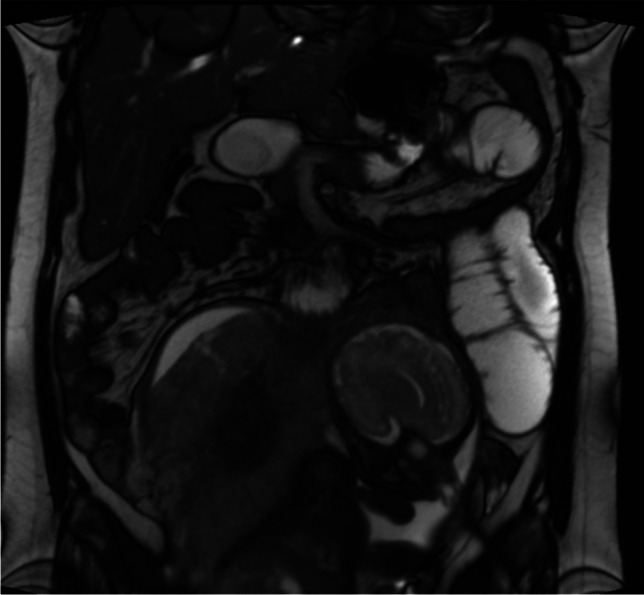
Intestinal obstruction visualized on MRI
